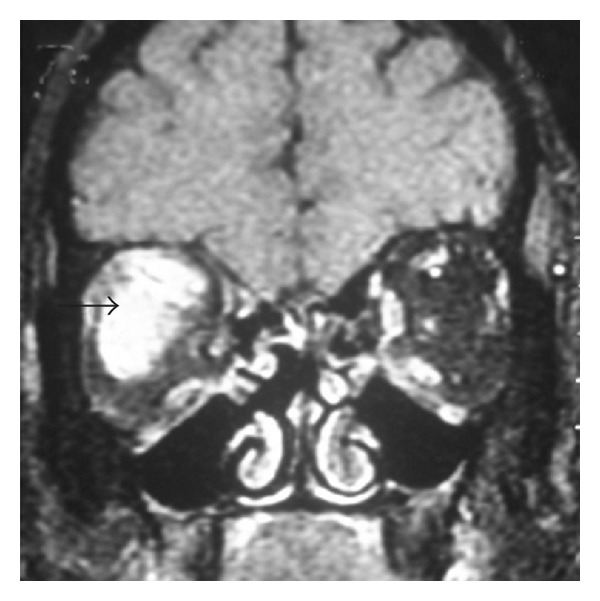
Coronal T1-weighted contrast image shows proptosis of the right globe. There is intense enhancement and enlargement of the right superior and lateral recti (black arrow).
